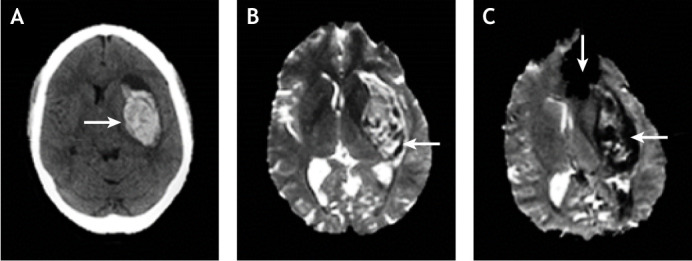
CT and MRI images showing intracerebral haemorrhage. (A-C) Images generated from CT (A), T2-weighted MRI (B) and GRE-MRI (C) scans, showing an intracerebral haemorrhage (horizontal arrows) at 2 h (CT) and 4.5 h (MRI) from symptom onset in an adult human. The vertical arrow in the GRE-MRI scan depicts the artefact caused by magnetic field inhomogeneity at the CSF/bone/air interface. Images reproduced with permission from Butcher and Davis (2009). These images are not published under the terms of the CC-BY license of this article. For permission to reuse, please see Butcher and Davis (2009). CSF, cerebrospinal fluid; CT, computed tomography; GRE, gradient recalled echo; MRI, magnetic resonance imaging.
